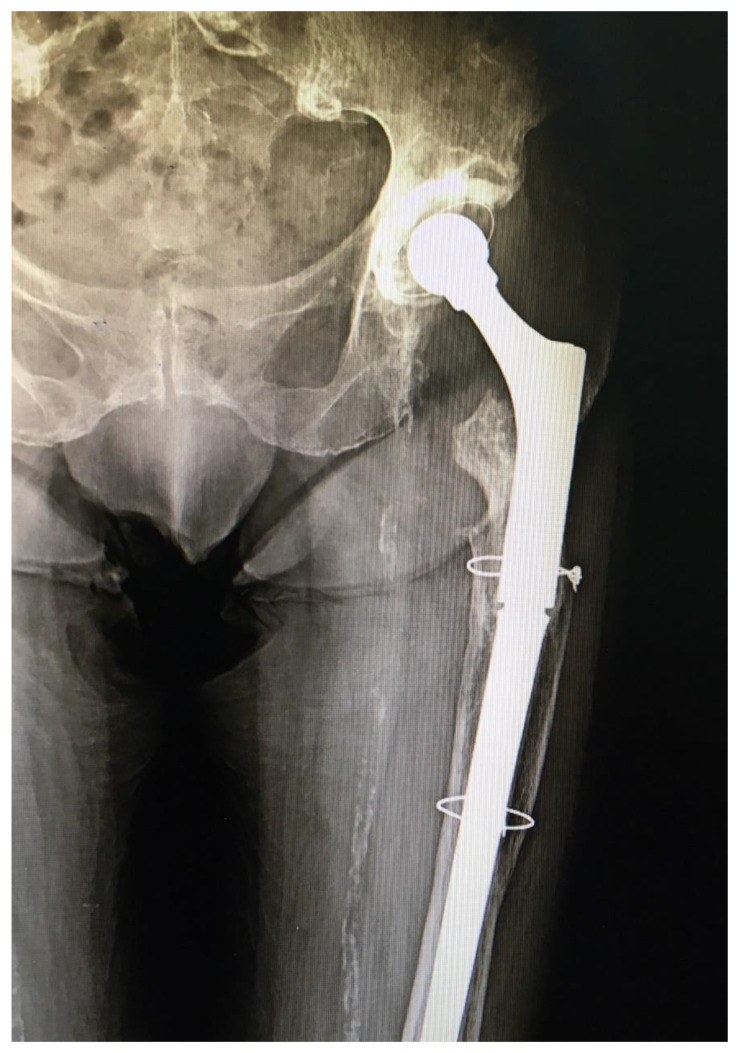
X-ray of the operated hip ten months after the surgery, showing a good position of the revision total hip endoprosthesis and no signs of instability.
